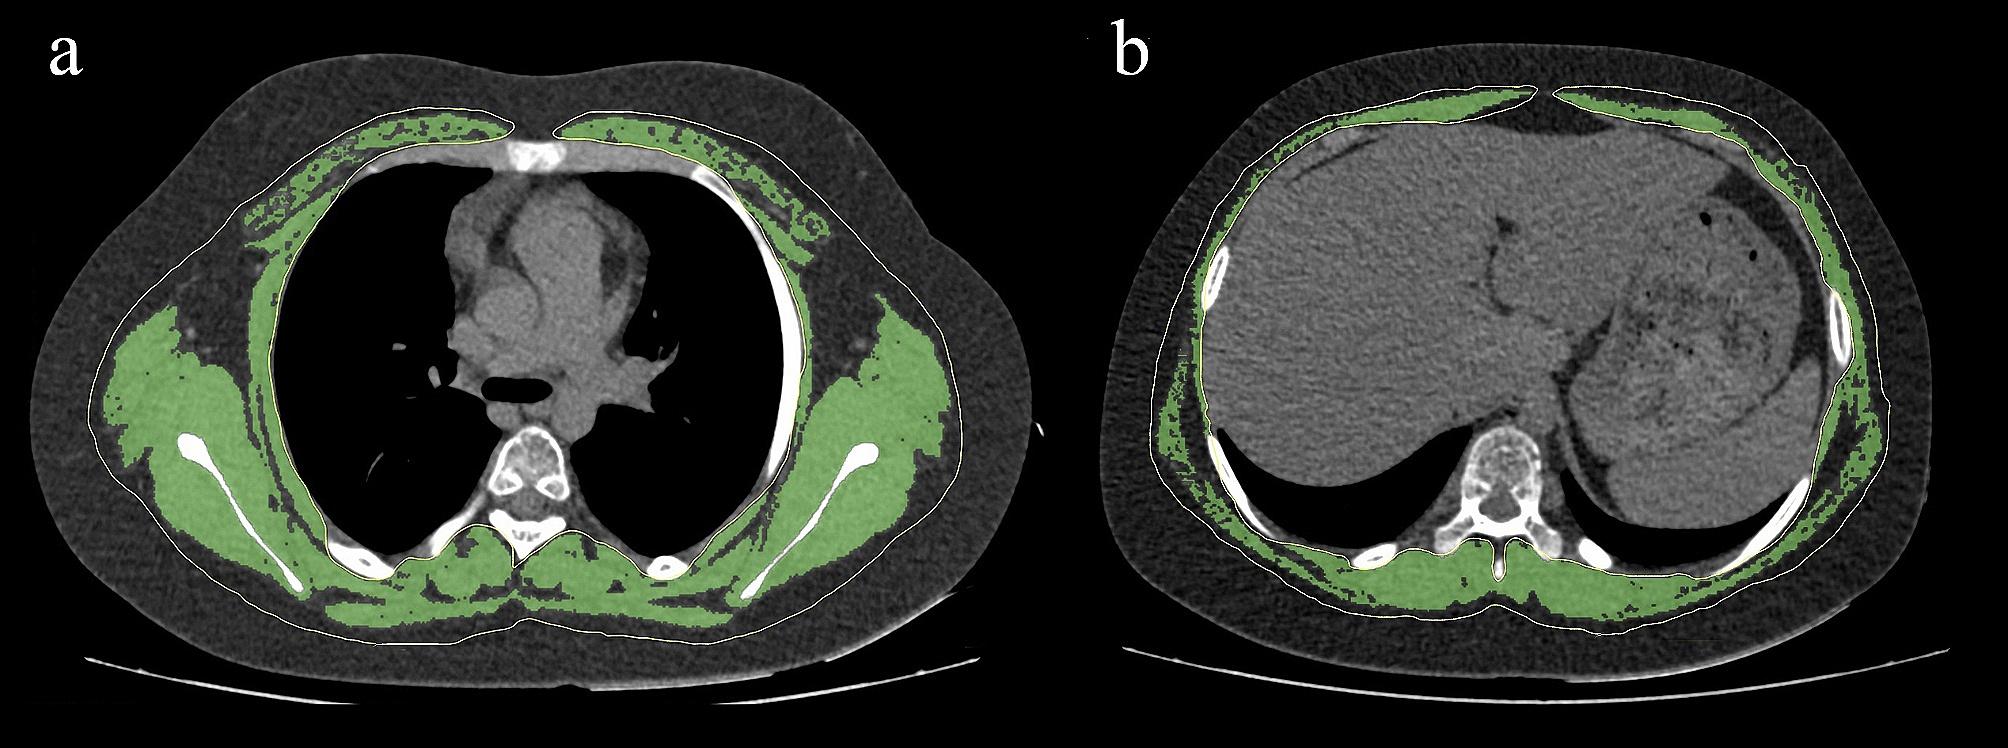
Measurement of cross‒sectional area and mean CT values of the trunk muscles. Measurement of cross‒sectional area and mean CT values of the trunk muscles at the level of the mid-T4 (a). Measurement of cross‒sectional area and mean CT values of the trunk muscles at the level of the mid-T10 (b)
